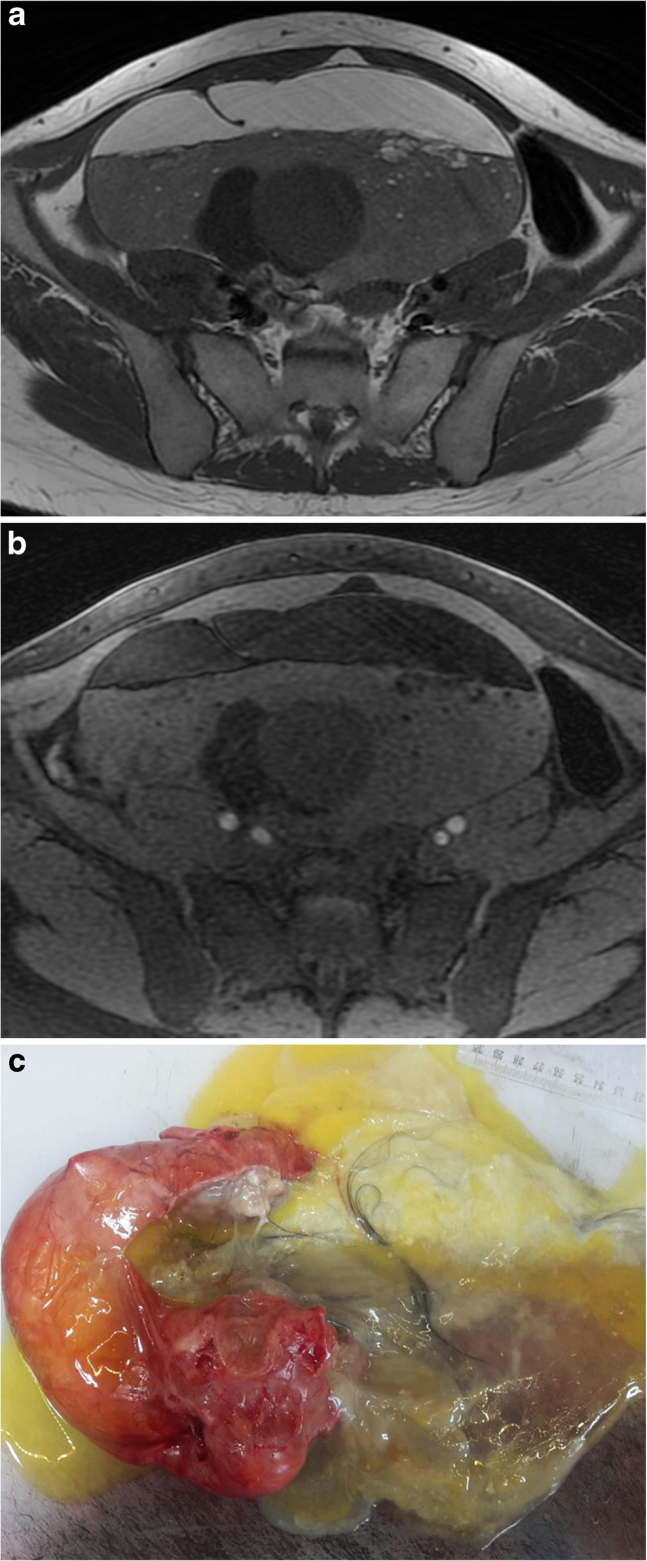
Axial T1-weighted MR image of a 31-year-old female with mature cystic teratoma (a) shows high signal intensity of the supernatant fatty layer creating an interface with a low signal intensity aqueous layer. Fat-saturated T1-weighted image (b) demonstrates a major fat component with decreased signal intensity in the supernatant layer and free-floating hypointense fat particles in the aqueous layer. A Rokitansky nodule with cystic components is seen in the posterior part of the cyst. Cut section of the tumour (c) shows yellowish sebaceous material leaking from the cystic lumen. Note the black hairs in that material
